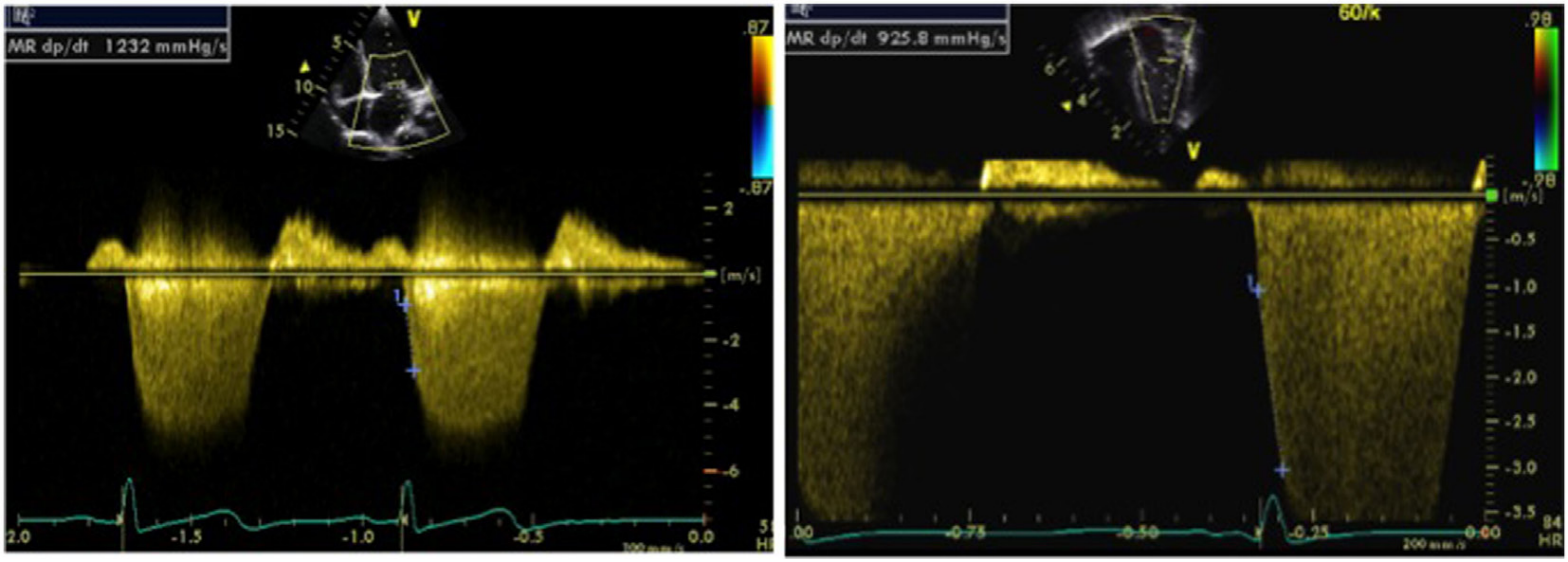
Doppler tracing from mitral regurgitation (MR) allowing measurement of the MR dP/dt max obtained between 1 and 3 m/s, on a normal patient (left image) and on a patient with left ventricular dysfunction (right image). An MR dP/dt max <500 mmHg/s is indicative of left ventricular systolic dysfunction. The same can be applied to tricuspid regurgitation (TR dP/dt max) but has to be measured between 1 and 2 m/s.
